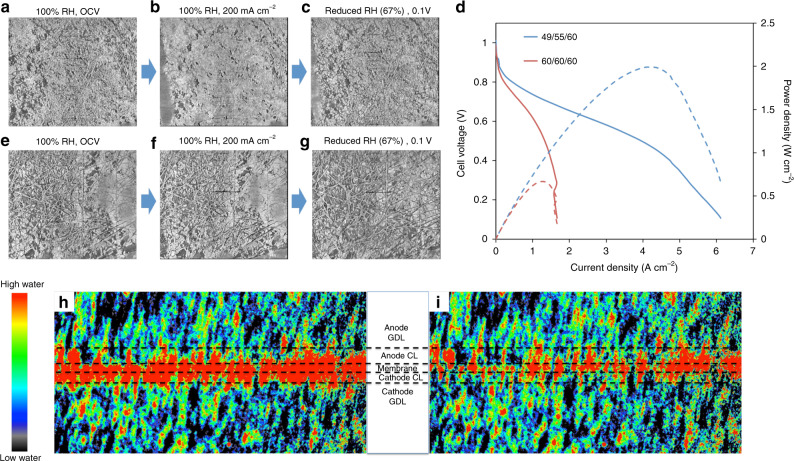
Dew point effects on electrode water and cell behavior. Operando micro X-ray computed tomography (CT) scans of the interface between the GDL and CL of (a–c) PtRu/C anodes and (e–f) Pt/C cathodes at conditions of: 100% RH at open circuit voltage (OCV), 100% RH at 200 mA cm^−2^, and reduced RH (67%) at 0.1 V, respectively. The H_2_/O_2_ flow rates were both kept at 0.2 L min^−1^. The cell temperature was kept at 28 °C (Polarization data for the operando cell is provided in Supplementary Fig. 5. d i-V (solid line) and i-power density curves (dashed line) at two different dew point configurations in 5 cm^2^ AMFCs. The membranes used in all tests were HDPE^5^. The 5 cm^2 ^ cells were tested at 60 °C with H_2_/O_2_ flows of 1 L/min. Operando neutron imaging of AMFCs at two different dew point situations: (h) The “full humidity” situation, which shows flooding inside the cell; (i) The “reduced dew points” situation, which shows drying out inside the cell. Anodes and cathodes used in the above three experiments had catalys loadings of 0.70 mg_PtRu_ cm^−2^ and 0.60 mg_Pt_ cm^−2^, respectively.
